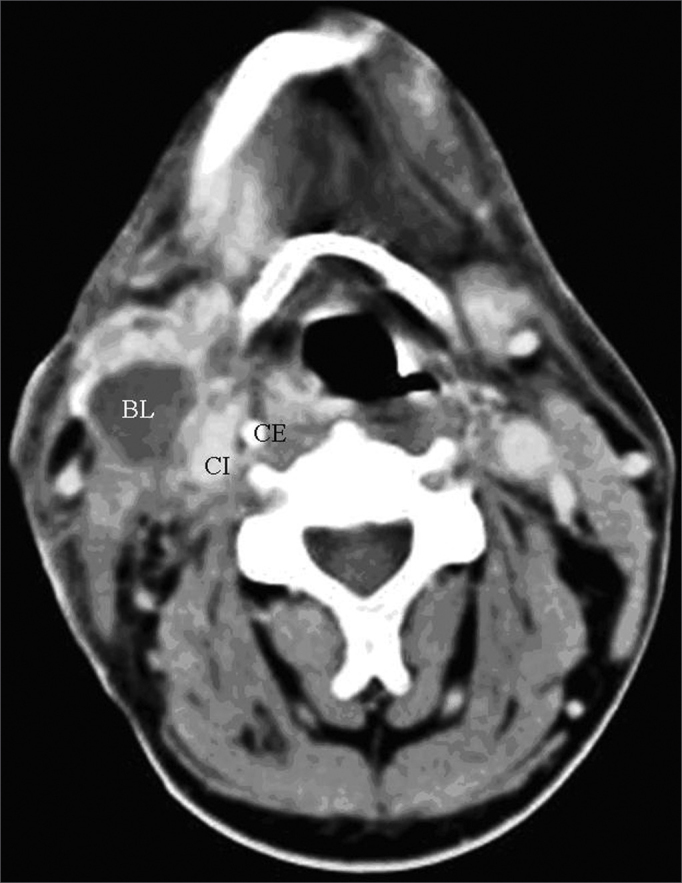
axial CT with endovenous contrast. – high jugular-carotid (level II) lymph node block (BL) to the left with a contact interface below 50% of the internal carotid artery circumference. CI: internal carotid artery; CE: external carotid artery.
